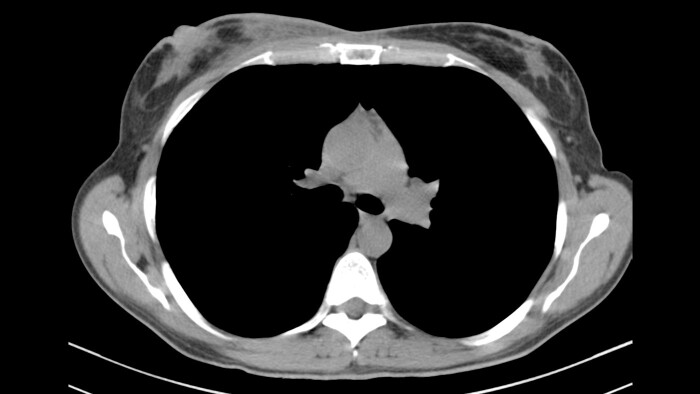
CT indicating heterogeneous enhancement and diffuse thickening of the skin of the right breast.
